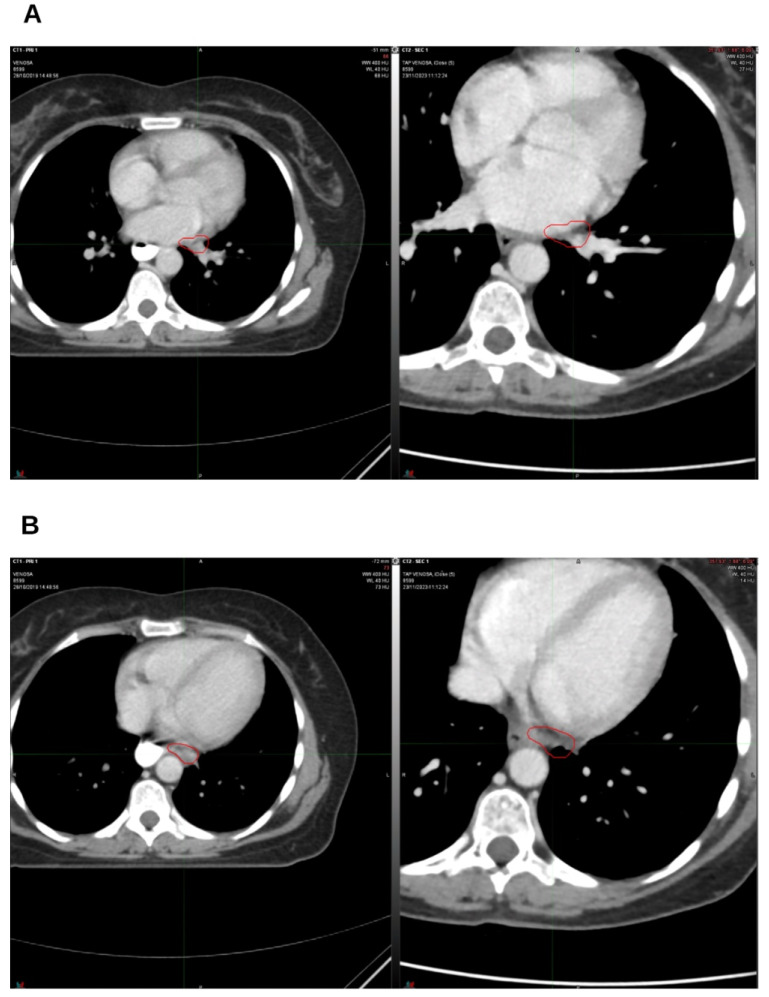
On the left, CT from October 2019, pre-radiation therapy treatment; on the right, CT from June 2022. (A) Hilar lymph node; (B) left para-aortic lymph node. The indicated region highlights the boundary of the treated target.
